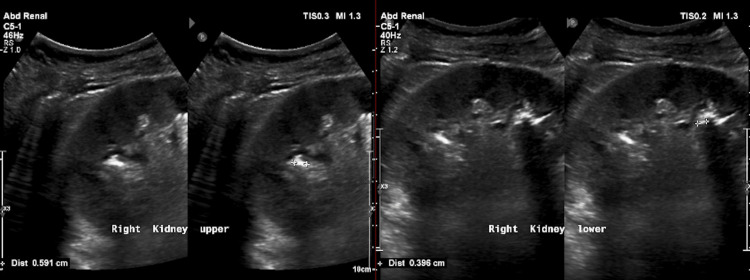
Ultrasound of the abdomen showing renal calculi in the right kidney measuring 5.9 mm
